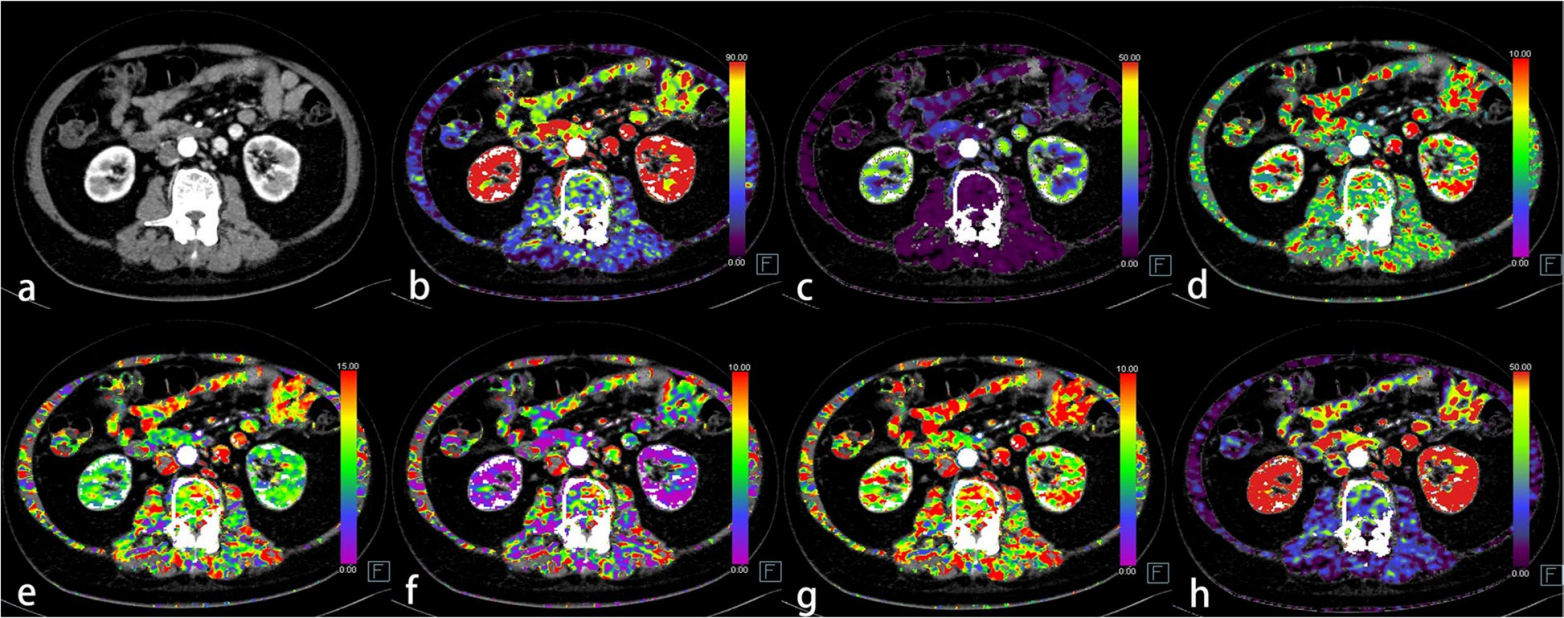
A 73-year-old male with normal bone mineral density, normal muscular mass, and high-grade sigmoid colon cancer (T_1_N_1_M_0_). a Axial abdominal contrast-enhanced CT image located at the central level of the vertebral body of lumbar 3. b The blood flow (BF) map of the CT perfusion (CTP). c The blood volume (BV) map. d The mean transit time (MTT) map. e The time to drain (TTD) map. f The time to start (TTS) map. g The time to maximum (T_max_) map. h The flow extraction product (FE) map
